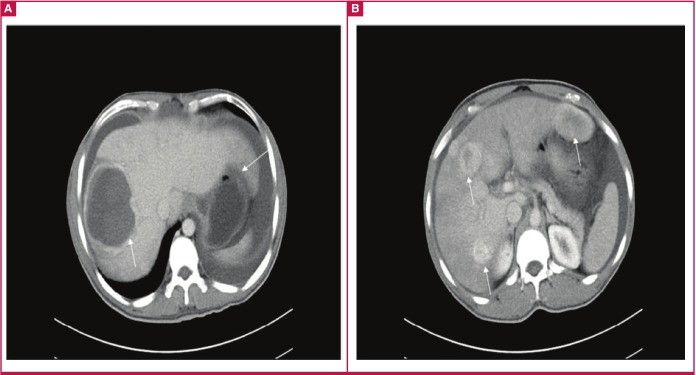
Patient 2. Computed tomography scan. Notice a large echogenic mass on the right lobe of the liver, and further multiple echogenic masses on both lobes (arrows).
